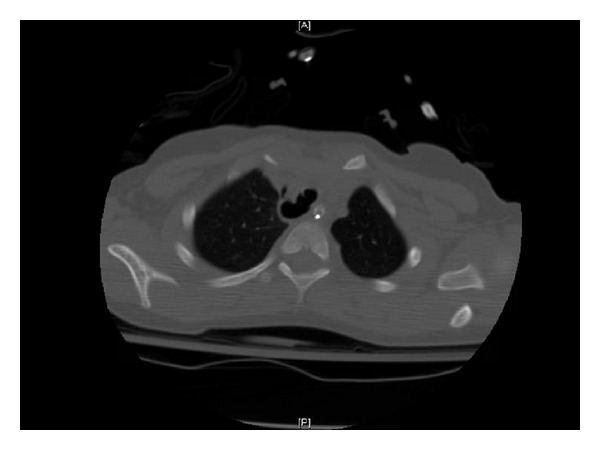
Hospital Day number 2. Noncontrast CT scan of chest. Extensive pneumomediastinum has diminished. There is a persistent right posterolateral tracheal wall defect/tracheal diverticulum.
